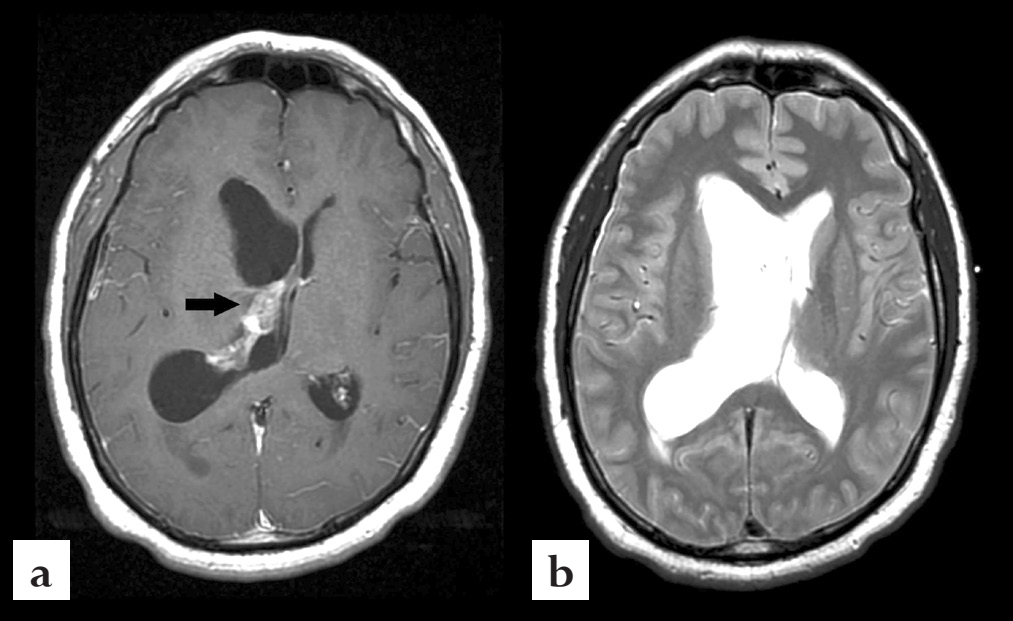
Case #2. (a): Axial T1 with contrast magnetic resonance imaging of the brain showing enhancement in right lateral ventricle without evidence of intraventricular cysts. (b): Axial T2 weighted magnetic resonance imaging of the brain showing right ventriculostomy catheter in the lateral ventricle. No intraventricular cysts were seen.
